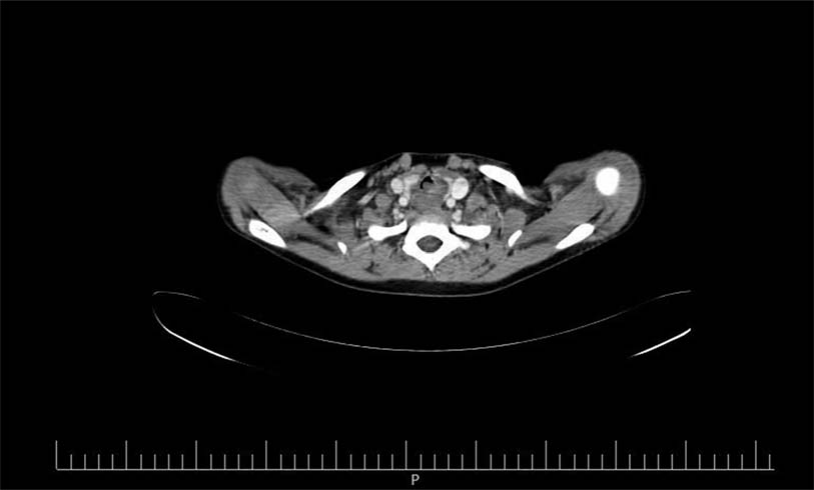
Demonstrate the neck CT scan of the patient demonstrating a vascular mass behind the larynx and cervical trachea.
